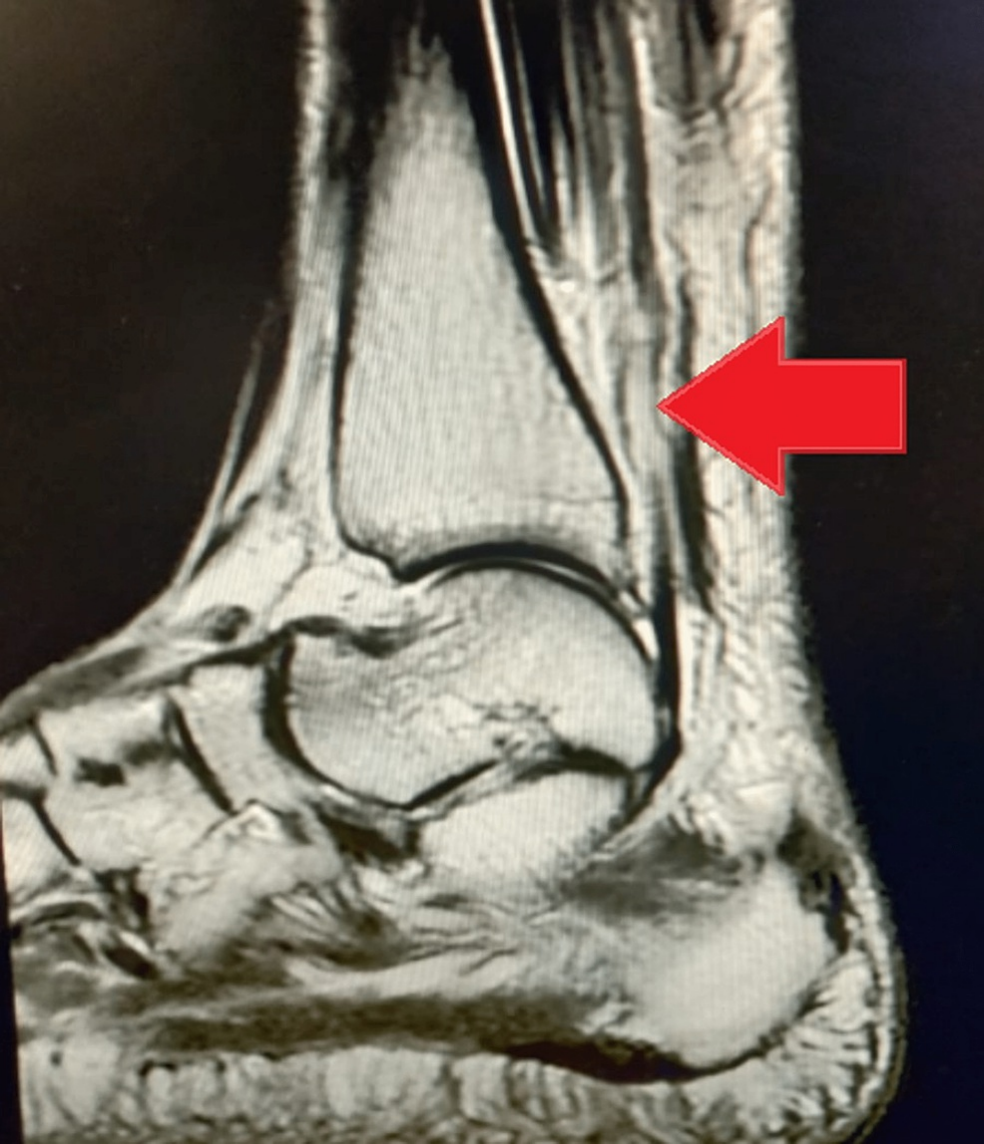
Pre-operative MRI showing large tendon defect (arrow)
